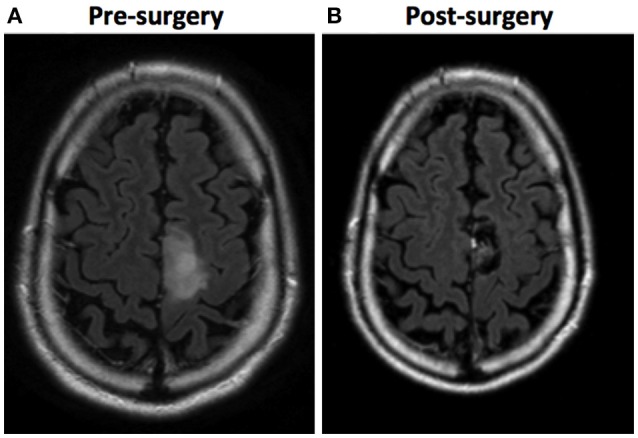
Example of an SMA lesion (in these images, left is right and right is left). (A) A FLAIR sequence MRI shows a tumoral lesion involving the left SMA in a 35-year-old patient. (B) A FLAIR sequence MRI 6 months after surgery shows a complete resection, achieved by performing awake brain mapping surgery. The pathology report was anaplastic oligodendroglioma.
